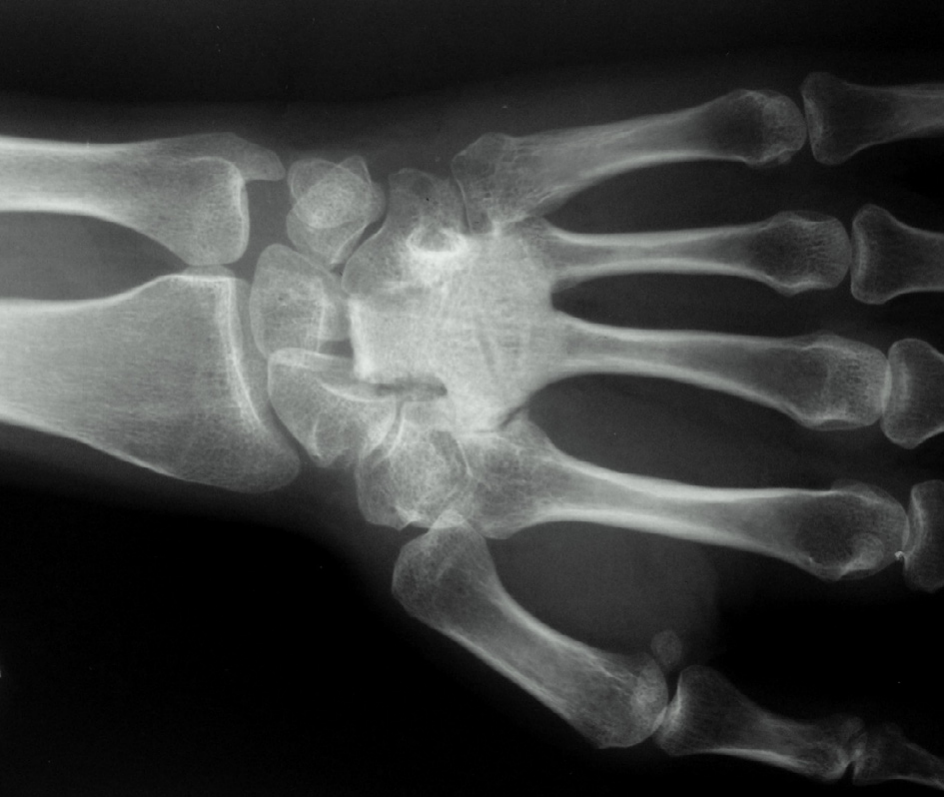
Roentgenogram of the operated wrist at 6-month follow-up.
